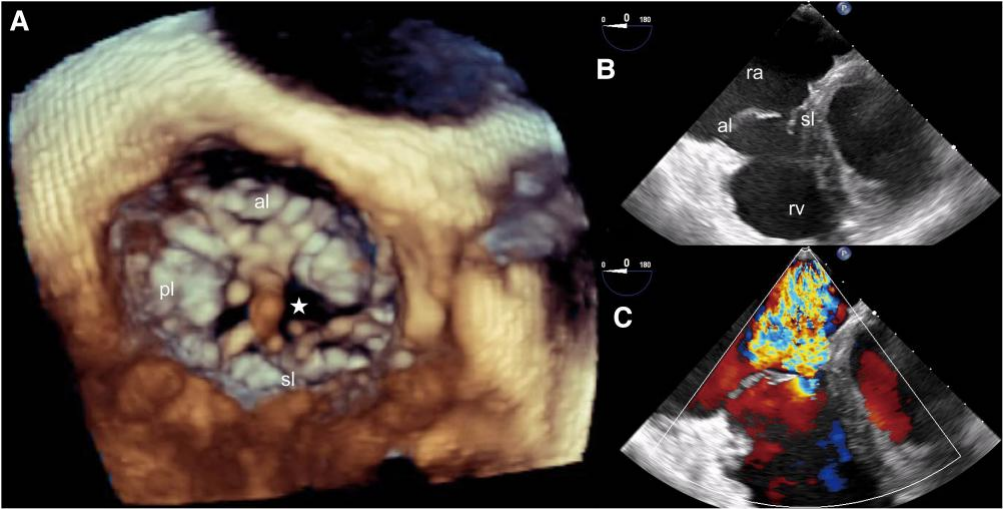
Tricuspid valve in the 3D-transoesophageal echocardiography (*A*) and in the 2D-transoesophageal echocardiography (*B* and *C* with colour Doppler) in the end-diastolic phase shows restriction of the septal leaflet, the regurgitant orifice (star), and the jet. Video clips are available in the supplementary material online. al, anterior leaflet; sl, septal leaflet; pl, posterior leaflet; ra, right atrium; rv, right ventricle.
